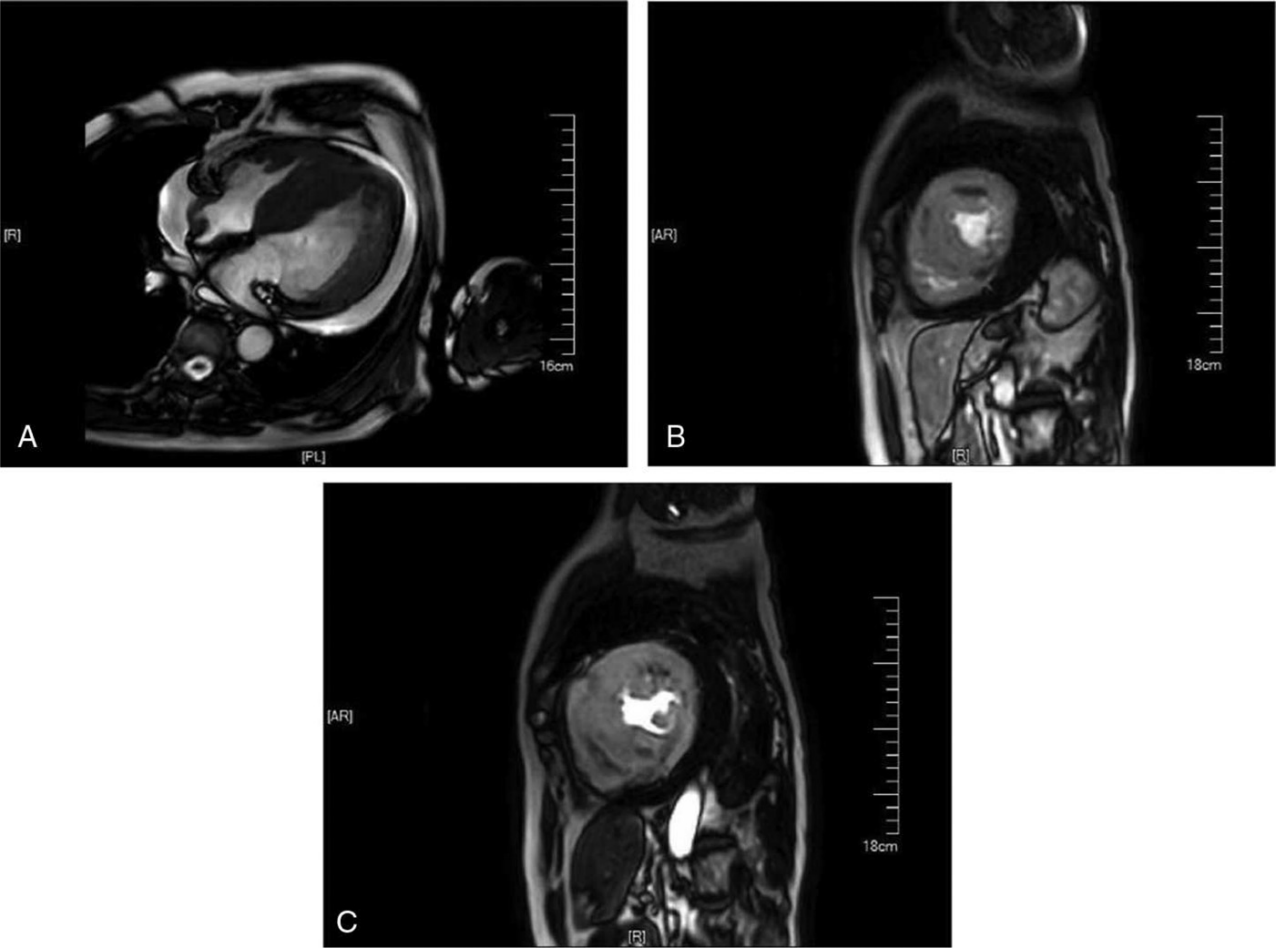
Cardiovascular magnetic resonance imaging: biventricular uneven hypertrophy, which was considered metabolic cardiomyopathy, with diffuse fibrosis of biventricular walls, apical thrombosis, and ischemic cardiomyopathy in the basal segment of the left ventricular lateral wall and left ventricular anterior wall.
